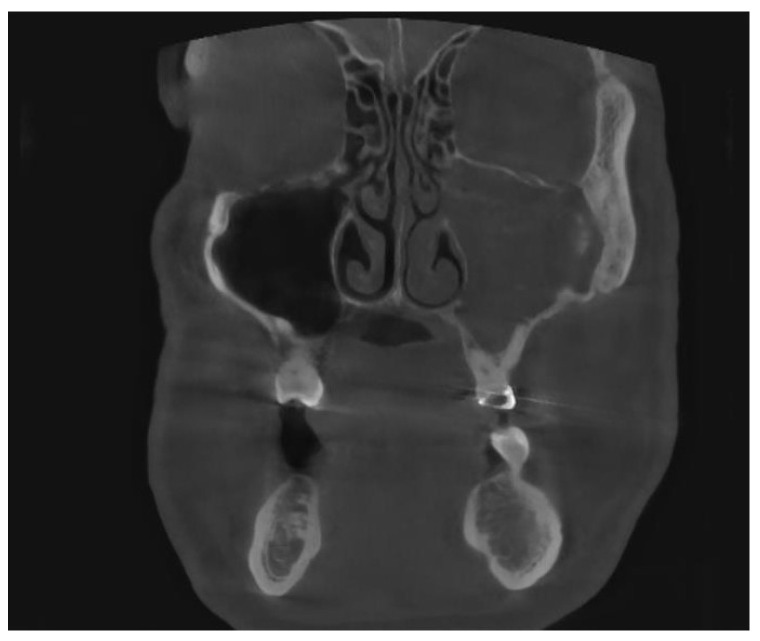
CBCT image of unilateral odontogenic maxillary sinusitis caused by periapical lesion. Maxillary sinus filled with secretions (coronal view).
